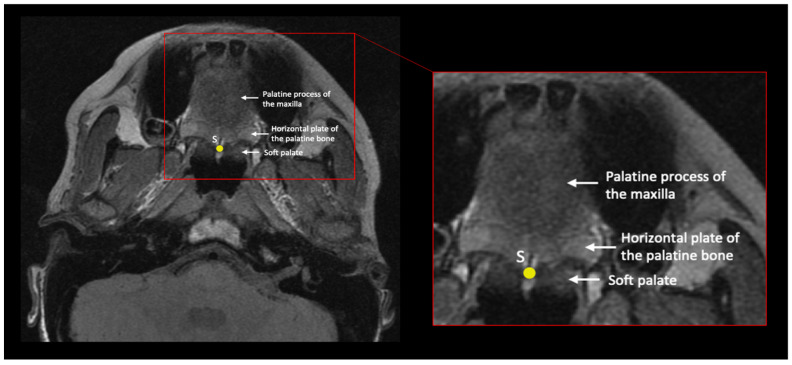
Oblique axial slice from an MRI scan depicting staphylion (S).
